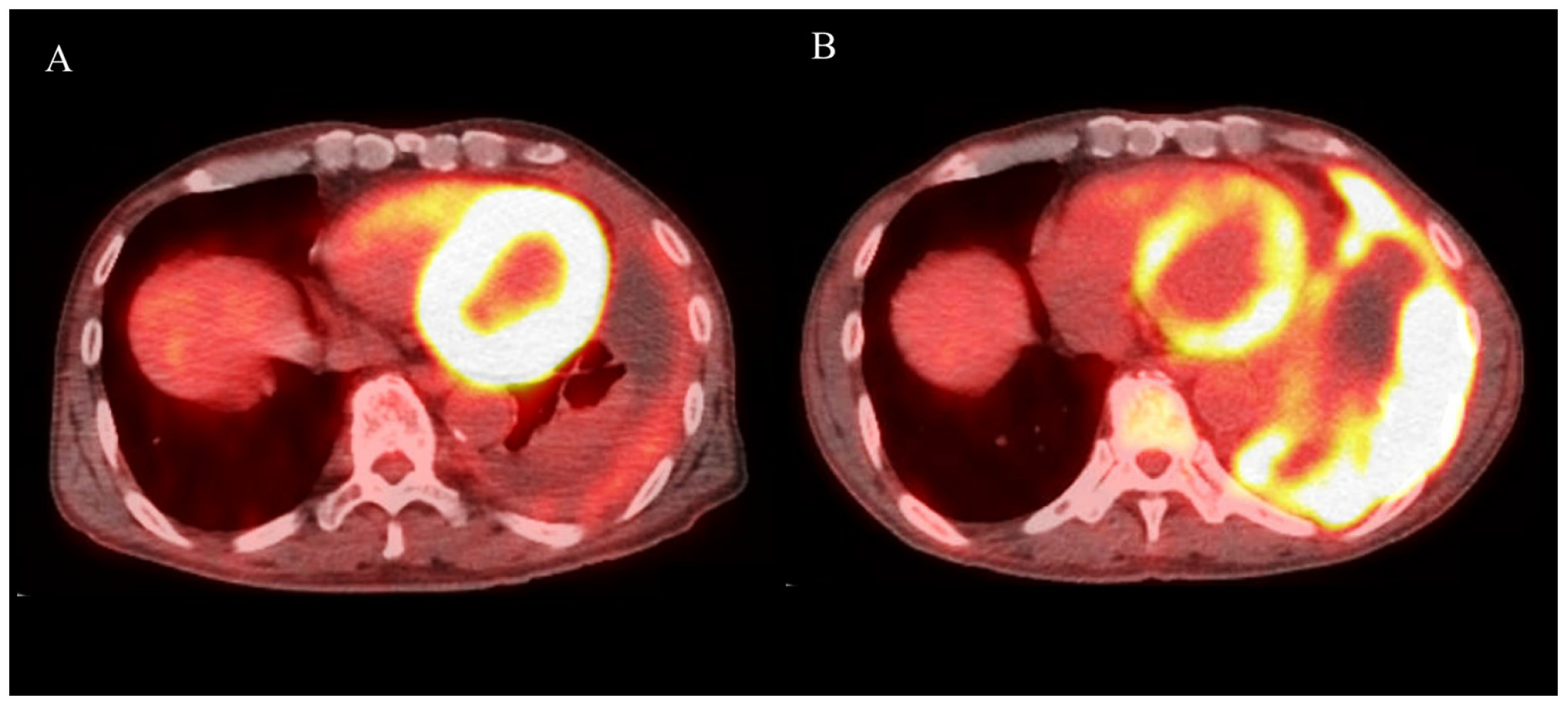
Positron emission tomography/CT images of Case 2. (A) After three cycles of nivolumab, the left pleural thickening and effusion improved, and ^18^F-fluorodeoxyglucose uptake decreased. (B) After nine cycles, the left pleural thickening and effusion worsened again. Other images are shown in our previous report (5).
